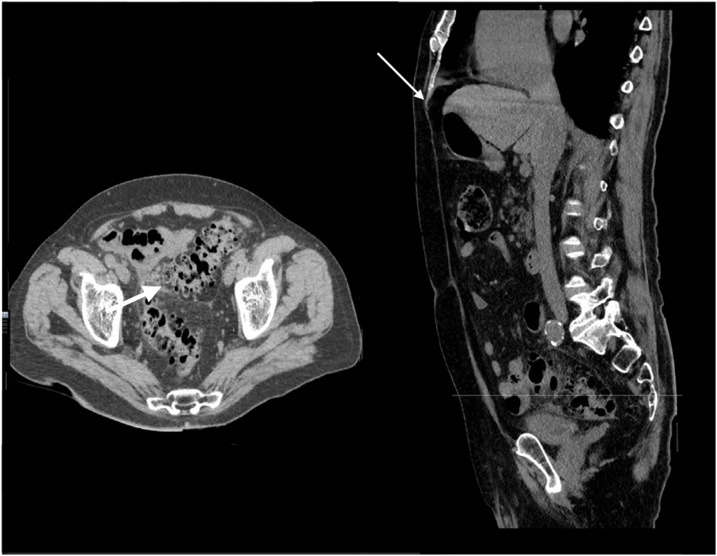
Preoperative abdominal CT scan showing complicated acute diverticulitis of the sigmoid colon (thick arrow) and free intraperitoneal air (thin arrow).
